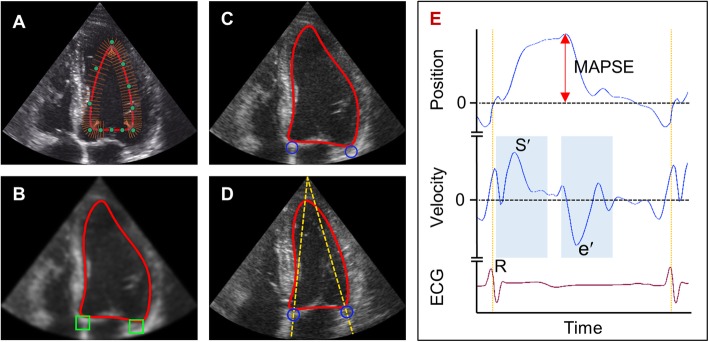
Acquisition of automatic measurements from apical 4-chamber colour tissue Doppler imaging recordings. a A deformable model is fitted to the left ventricle by edge-detection on B-mode frames. b Search for the mitral annulus. The brightest pixel within each green box is assumed to be from the annulus. c and d The positions of the tracked points (blue circles) are updated by trapezoidal integration of the Doppler velocity signal, and move straight towards the probe origin (yellow, dashed lines). e Position- and velocity data from the tracking points are analysed. The mitral annular plane systolic excursion (MAPSE) is estimated by finding the difference between the start and peak position. The mitral annular peak velocities (S′ and e′) are found within pre-defined time intervals (light blue regions)
